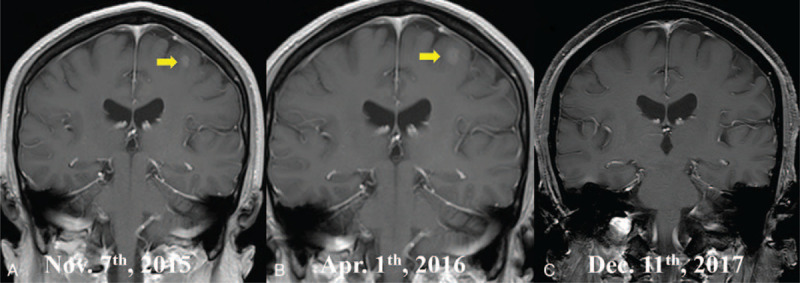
The brain magnetic resonance imaging. (A) A solitary BM was indicated before the therapy (labeled by the yellow arrow); (B) The intracranial lesion maintained stable after 3 cycles of pemetrexed/carboplatin (labeled by the yellow arrow); (C) A complete remission of the BM was demonstrated 2 yr after the combination treatment using pemetrexed and anlotinib for 3 cycles and maintained anlotinib monotherapy for 1 yr. BM = brain metastasis.
